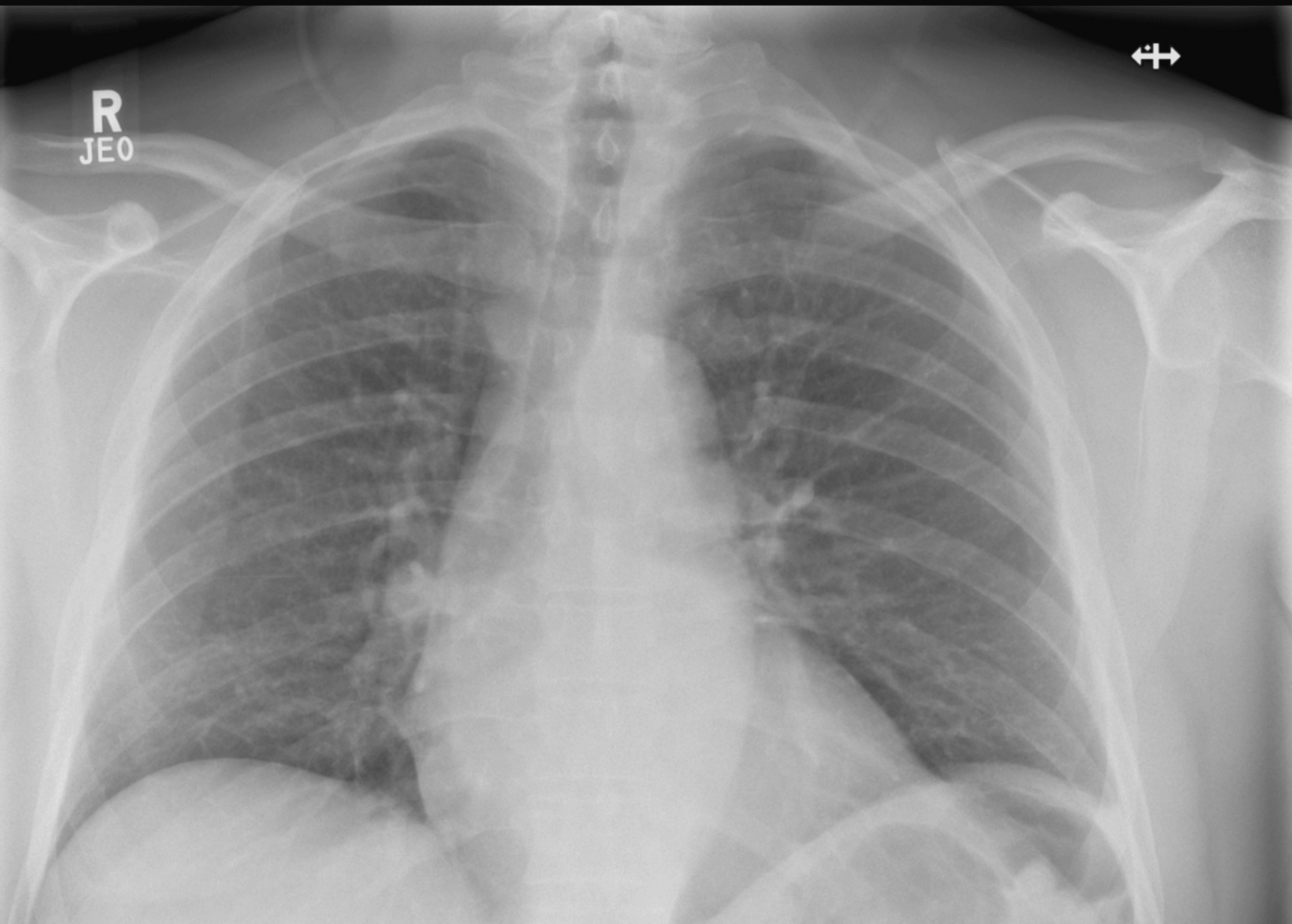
Follow-up chest radiograph obtained three days after the APE episode, demonstrating interval resolution of pulmonary congestion APE, acute pulmonary edema
